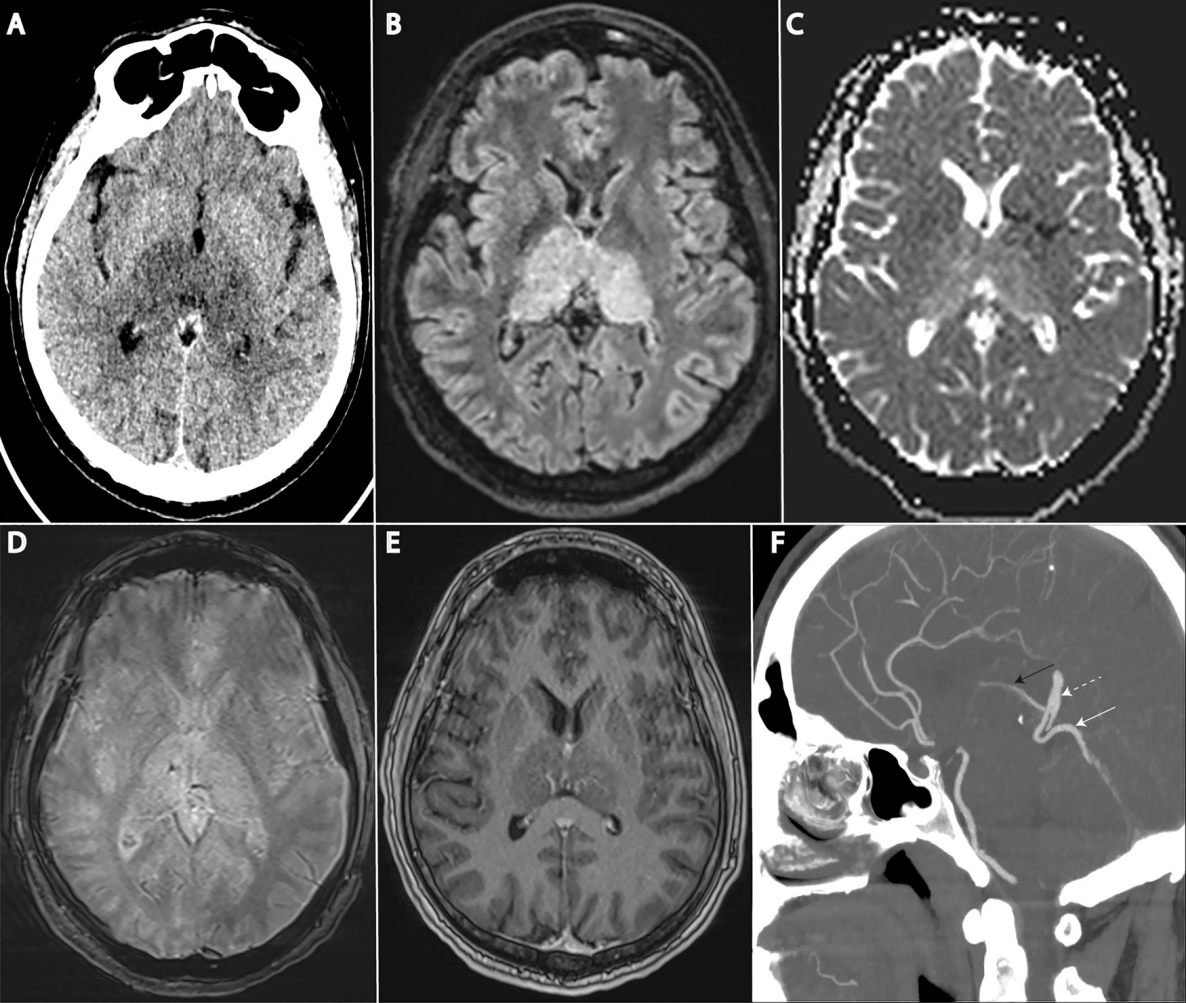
Noninvasive Imaging A: Plain CT scan showing bithalamic symmetrical hypodensities B: Baseline MRI showing bithalamic FLAIR hyperintensities C: Diffusion MRI depicting increased bithalamic apparent diffusion coefficient (ADC) D: Punctuate hemorrhage in right thalamus on T2* E: Gadolinium enhanced sequence showing small foci of bithalamic patchy enhancement F: Arterialized superior vermian vein (white arrow) draining into vein of Galen (dotted white arrow) and subsequently into internal cerebral vein (black arrow).
